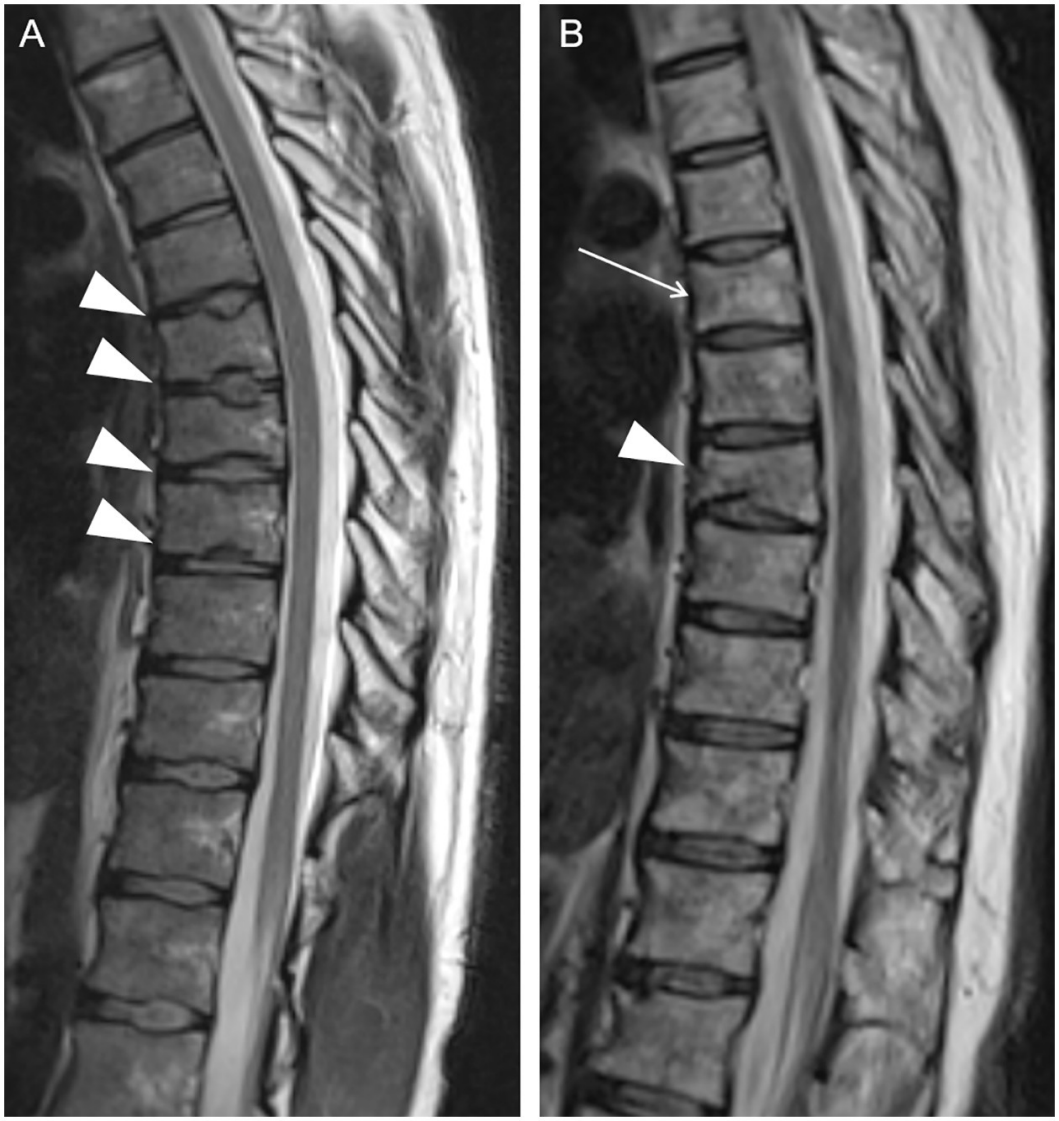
Representative spinal magnetic resonance imaging (MRI) showing vertebral changes on T2-weighted (T2W) sagittal images. Several adjacent flat thoracic vertebrae with end-plate irregularities are demonstrated on T2W image of a male aged 31 years (A, arrowheads). T2W image of a female aged 43 years demonstrates slightly compressed Th6 vertebra, Genant’s grade 1 (B, arrow) and vertebral fracture of Th8, Genant’s grade 2 (B, arrowhead).
